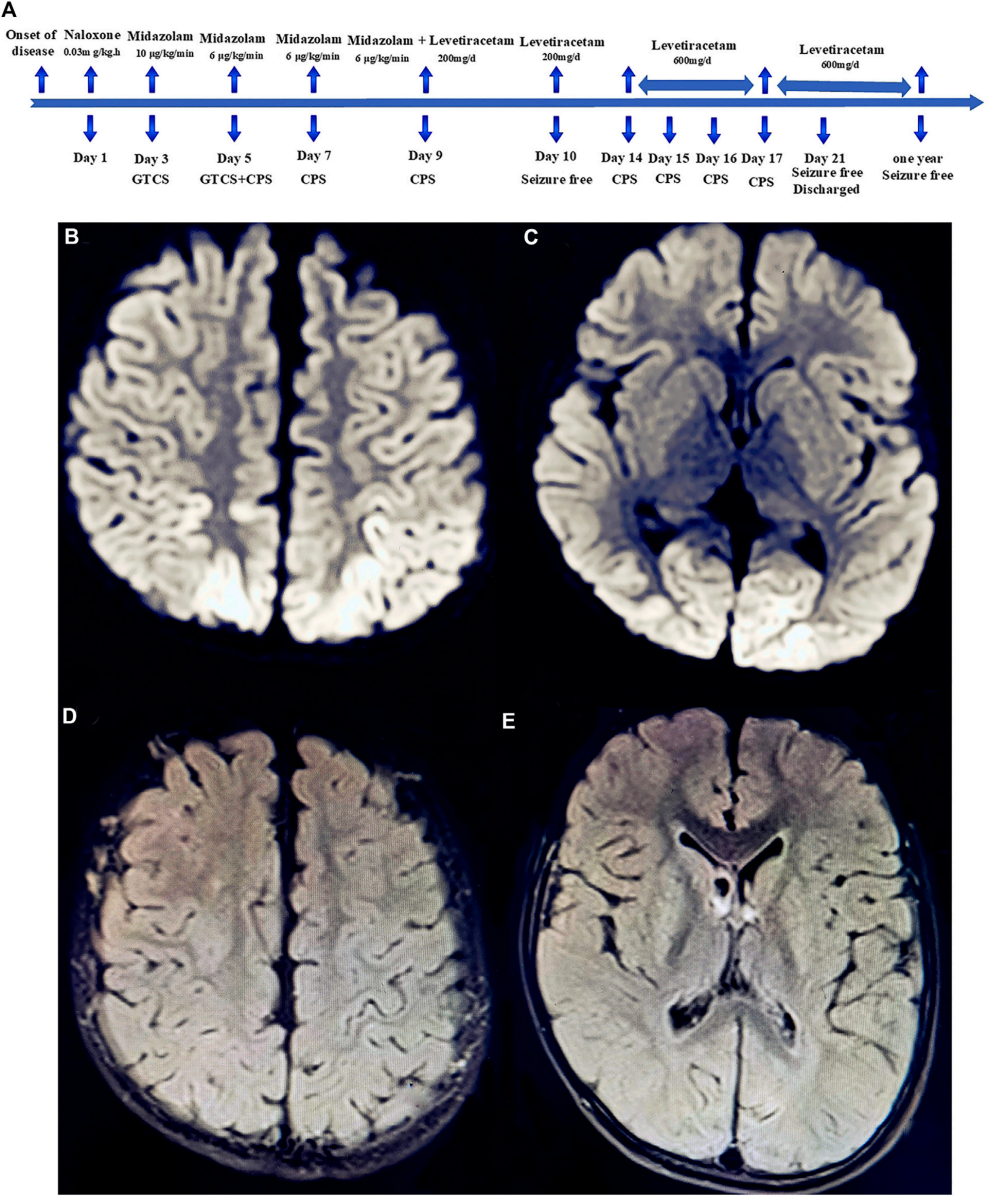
Timeline of the patient’s clinical course and outcome (A). Diffusion-weighted magnetic resonance imaging (DW-MRI) of the head showed abnormal high signal intensity in the bilateral parietal lobe (B), anterior wedge, and occipital lobe (C). Head MRI-DWI re-examined after 1 year of follow-up revealed that the abnormal signal intensity of brain gray matter disappeared completely (D,E).
